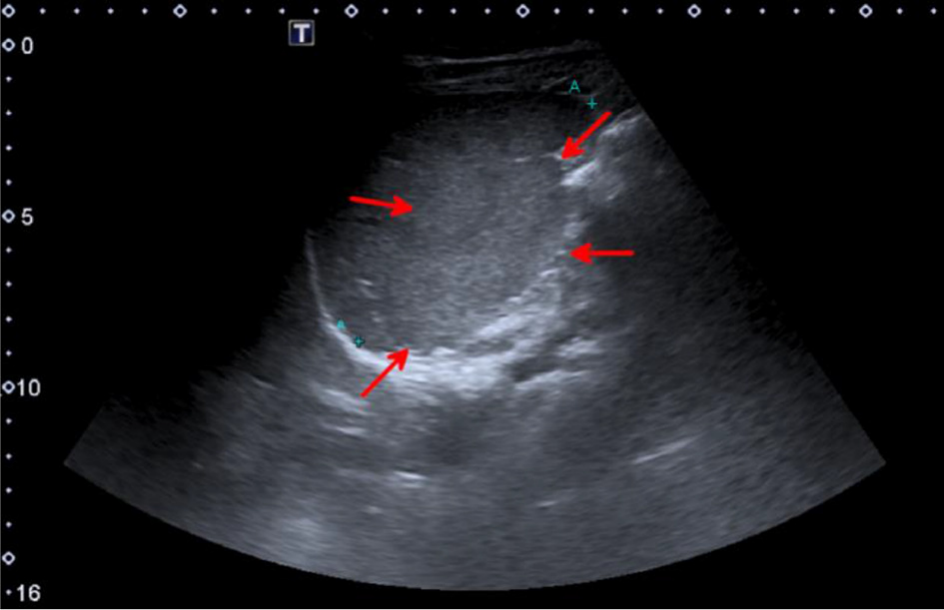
Long axis ultrasonography image of the spleen. The medial (internal) lobe of the spleen (red arrows) seen as a smooth ill-defined bulge from the splenic hilum. The medial lobe isoechoic to the rest of the spleen.
